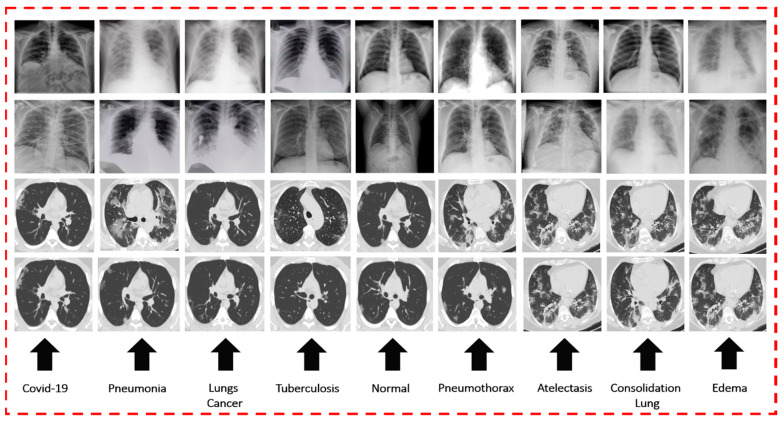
CT scan and CXR sample images of nine chest diseases.
